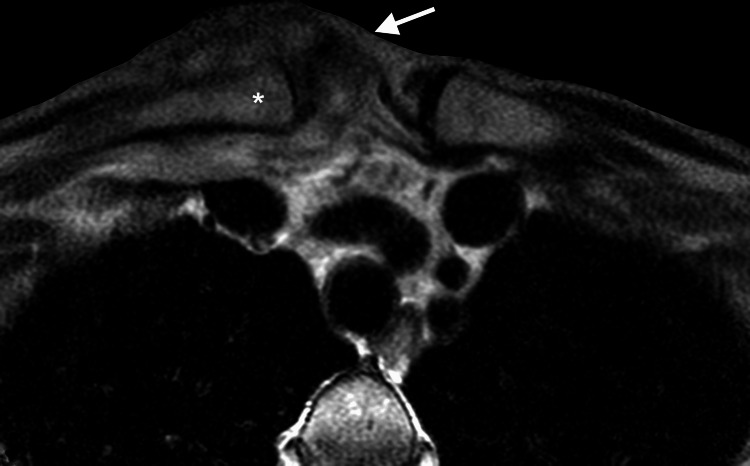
Axial MRI of patient's right sternoclavicular joint. Axial MRI scan showing the patient's right SCJ infection (arrow). The asterisk indicates the medial aspect of the clavicle, which had mild heterogeneity suggestive of acute osteomyelitis. (Motion artifact degraded the quality of this image; additional imaging was not done.) SCJ: Sternoclavicular joint.
